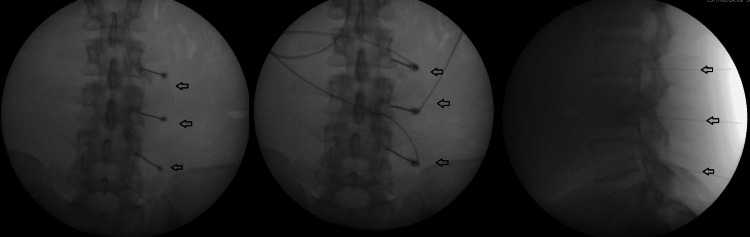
X-ray-guided imaging of right L2-L4 dorsal root ganglion Left and Middle images are anterior-posterior views with a radiofrequency ablation probe; Right image is a the lateral view.
